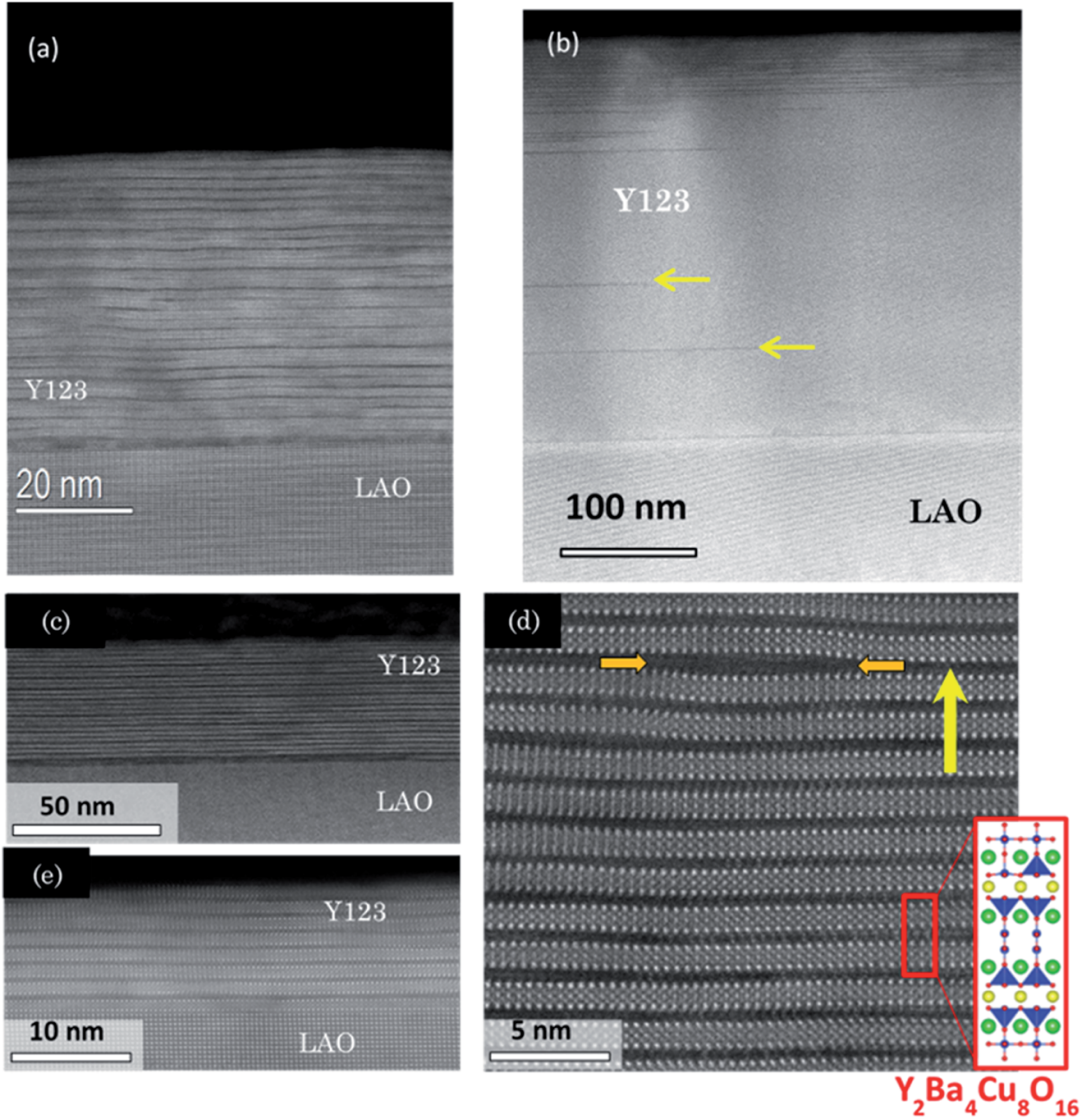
(a) STEM image of a FH Y123 film with a thickness of 45 nm where Y248 layers (black horizontal lines) are seen to be distributed inhomogeneously along *c*-axis; (b) pristine 250 nm Y123 thin film grown by the CTA process at 810 °C. The yellow arrows indicate examples of Y248 intergrowths; (c) *Z*-contrast low magnification STEM image of a 50 nm film with a high concentration of Y248 intergrowths; (d) a higher resolution STEM image of the 50 nm ultrathin film shown in (c) focusing on the bulk of the film. The inset shows the Y248 structure and its association with the observed planes; (e) *Z*-contrast STEM image of a 10 nm film. The horizontal dark stripes that cross the image are due to the formation of Y248 intergrowths. The yellow arrows in (e) show an intergrowth with an additional CuO layer resulting in the local formation of the Y125 structure.
